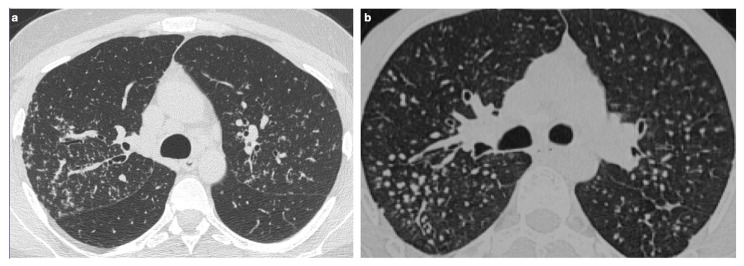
An example of typical sarcoidosis characterized by the presence of diffuse micronodules with perilymphatic distribution (a). The HRCT of a patient with occupational exposure to silicium is characterized by the presence of diffuse nodules predominantly in the upper lobes (b).
